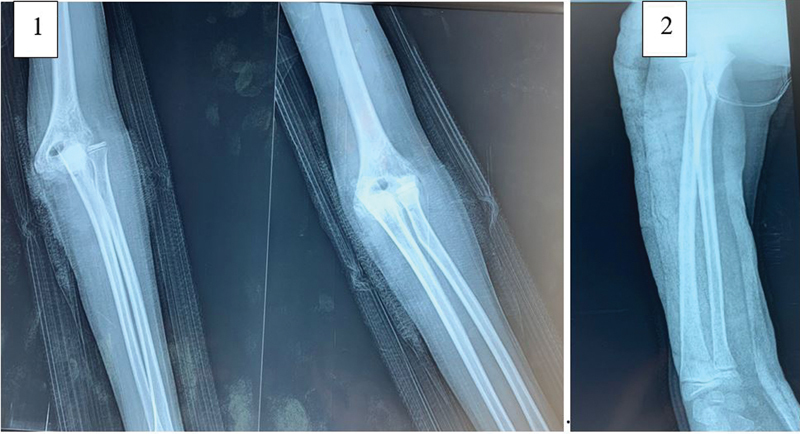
Standard preoperative radiograph showing posterolateral elbow dislocation.
